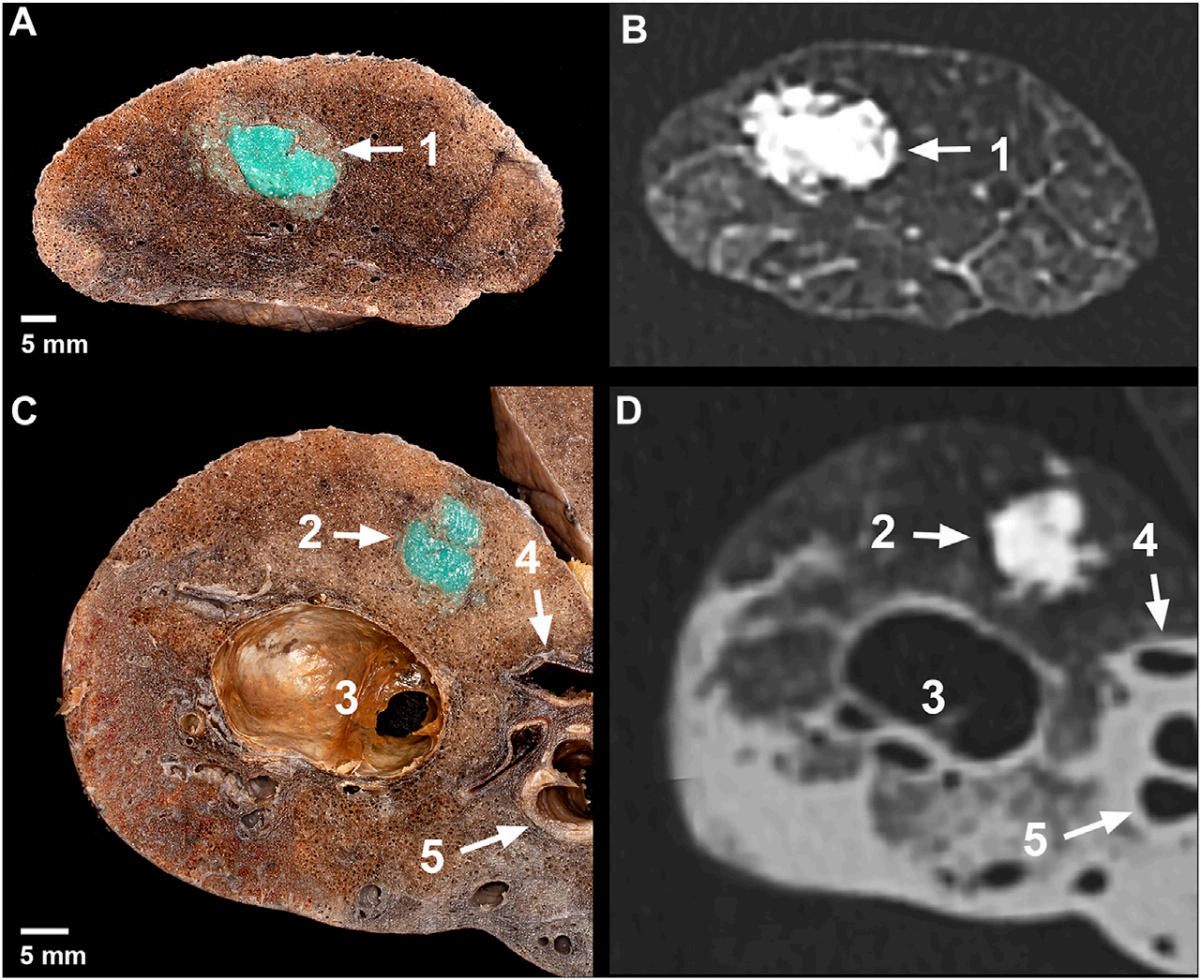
Macroscopic photographs and CT images of the artificial tumors. Axial slice (A) and its CT image (B) in the apical region of a right lung– 1: apical lesion; Axial slice (C) and its CT image (D) in the middle lobe of a right lung– 2: 1.0 ml peripheral lesion with spiculated formation, 3: emphysematous bulla, 4: medial segmental artery, 5: lateral segmental bronchus.
